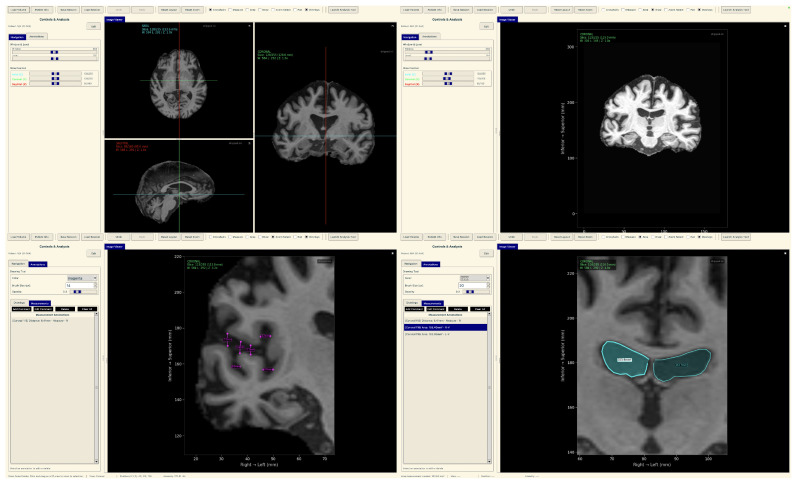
Neuroimaging slice viewer interface demonstrating MRI visualization capabilities.
